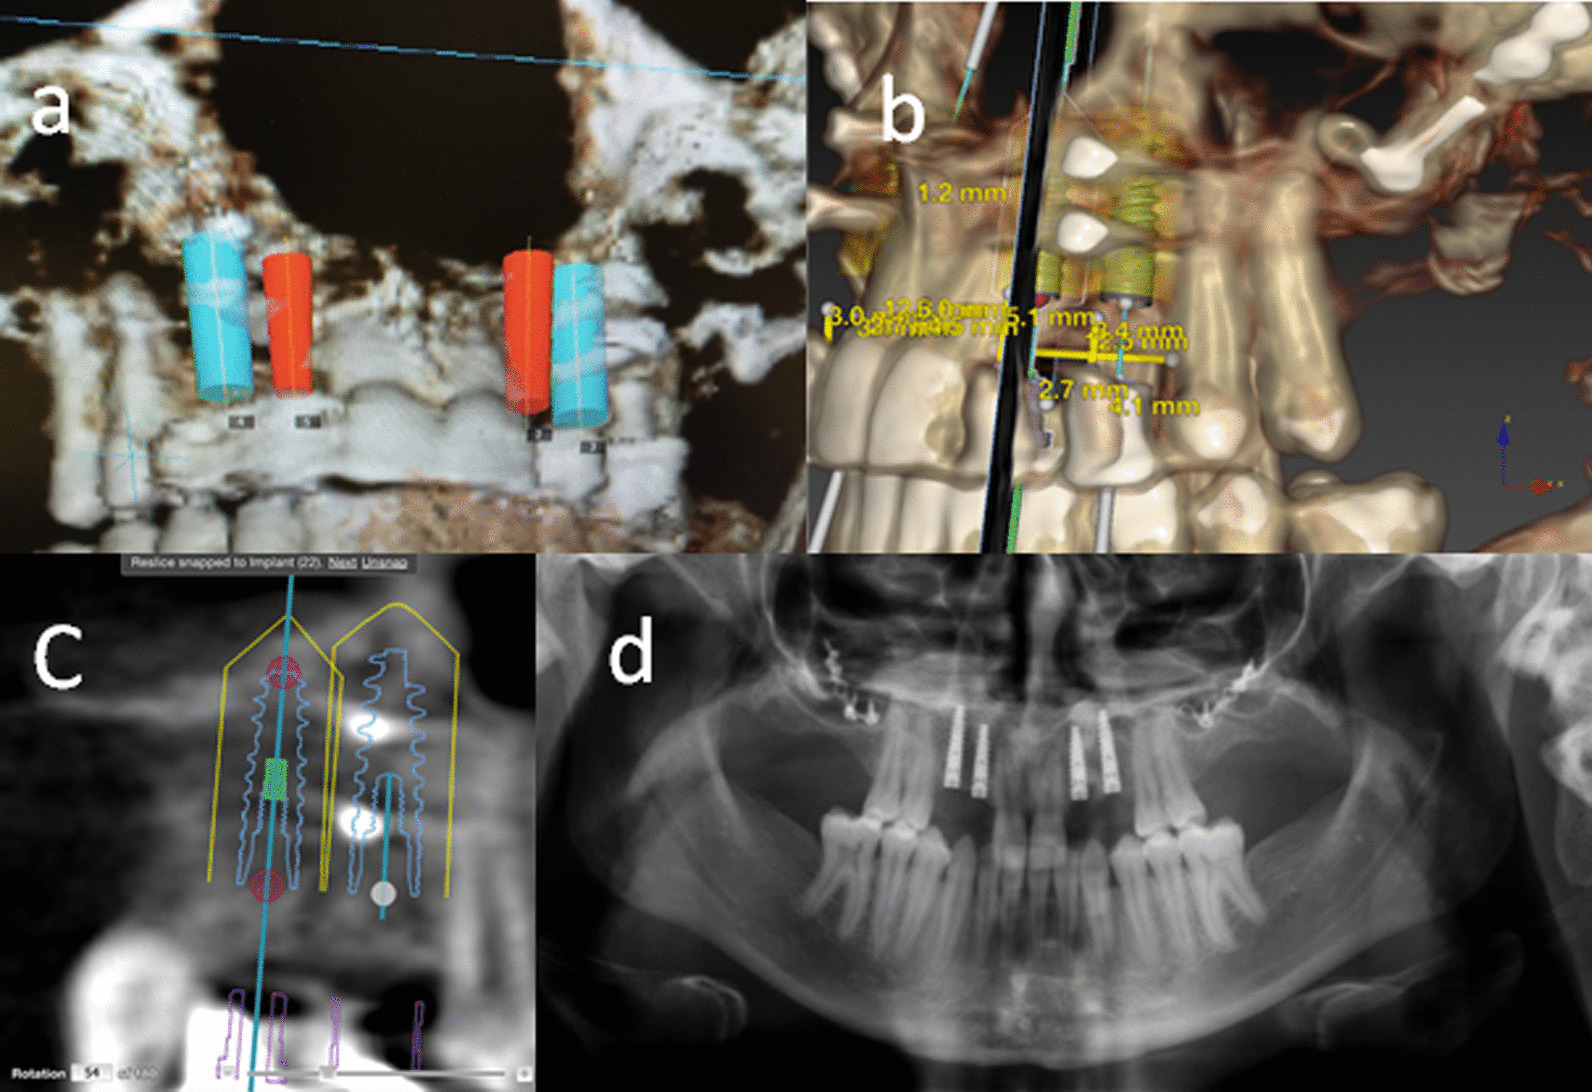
Implant placement: a–c Implant placement planning using CBCT with radiographic guide. d Panoramic radiograph showing implant installation
